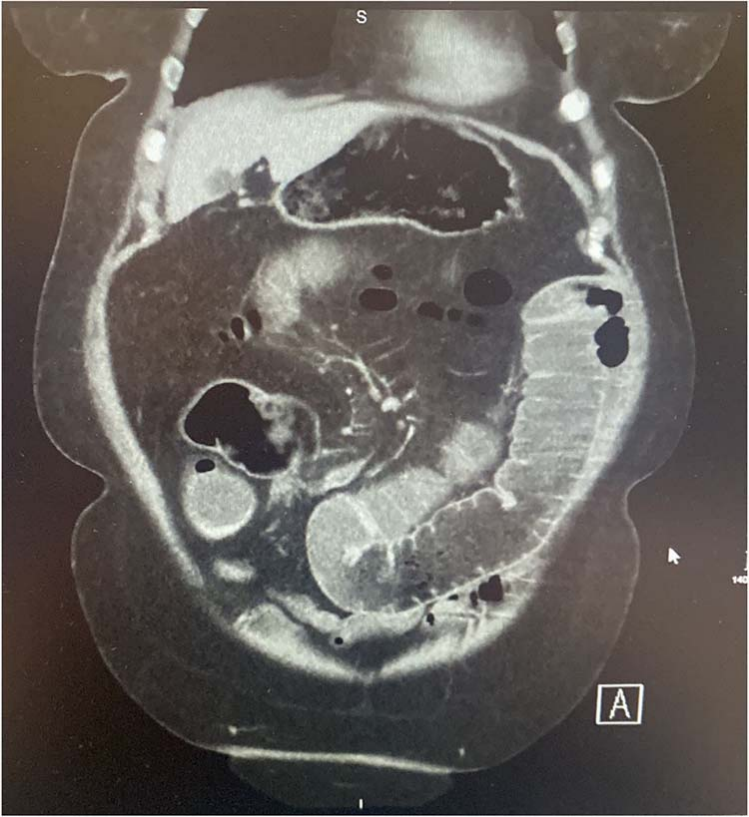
Coronal section of contrast CT AP demonstrating small bowel intussusception with proximal dilation and associated filling defect.
